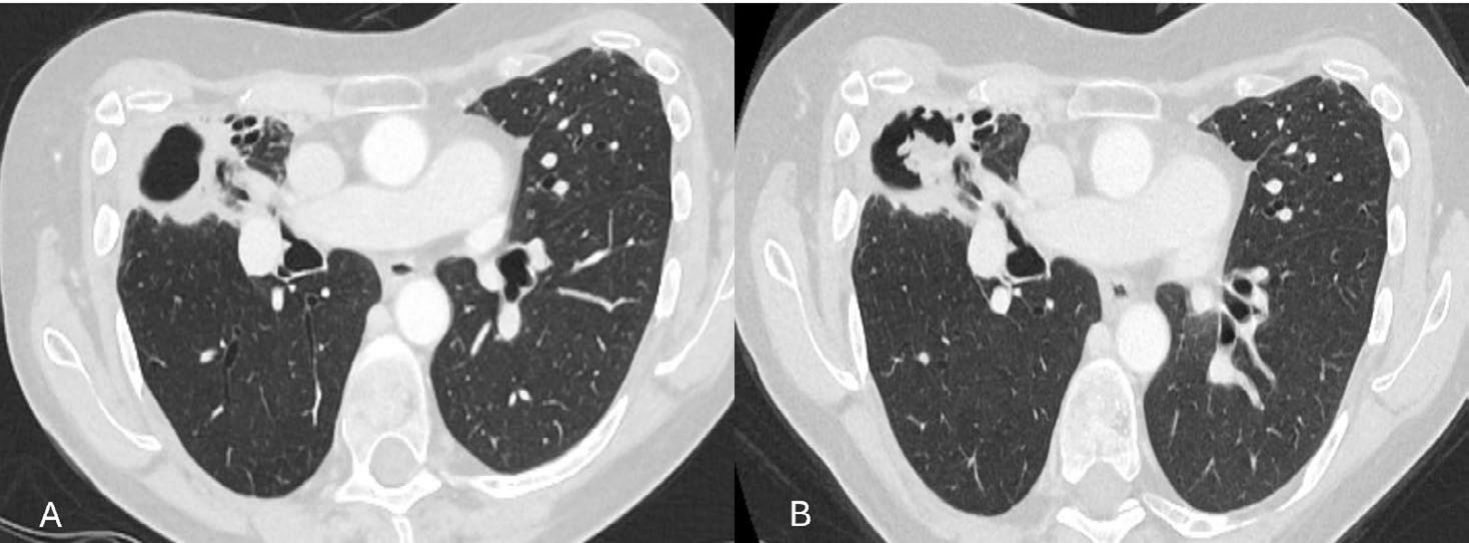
56‐year‐old woman with a past medical history of asthma, bronchiectasis and previous thoracic surgery for pectus excavatum. She was previously treated with voriconazole for 2 years and discontinued therapy due to clinical stability. (A) CT scan at end of treatment. (B) One year after stopping antifungal treatment, she reported worsening dyspnoea and a productive cough. A CT scan demonstrates a new fungal ball within the right upper lobe cavity.
